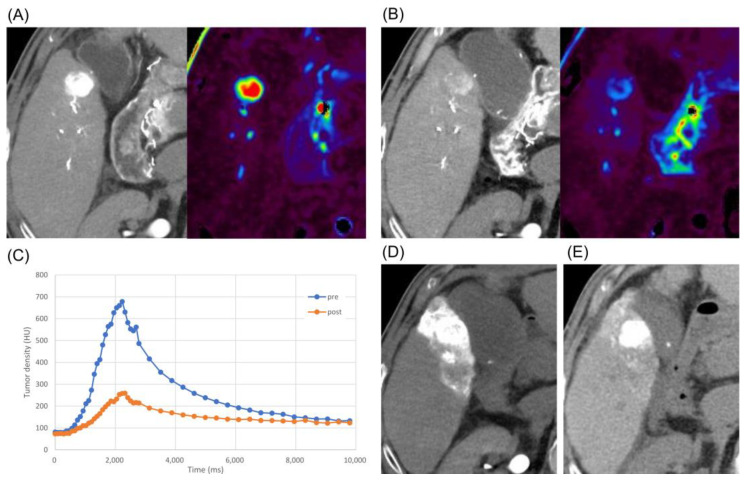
Case 1: quantification of tumor vascularity. (A) 4D-CTHA and perfusion map just before the initiation of lenvatinib. Arterial blood flow (ABF) to the tumor was 487.9 mL/min/100 mL. (B) 4D-CTHA and perfusion map after lenvatinib administration for 7 days. ABF decreased to 139.5 mL/min/100 mL, which was 28.6% of the value before lenvatinib administration. (C) Time intensity curves pre- and post-lenvatinib: a decrease in intratumoral density was observed post-treatment compared to pre-treatment. (D) Plain CT immediately after cTACE: lipiodol deposited well in the tumor. (E) Plain CT after 6 months: persistent complete lipiodol retention was seen in the tumor.
